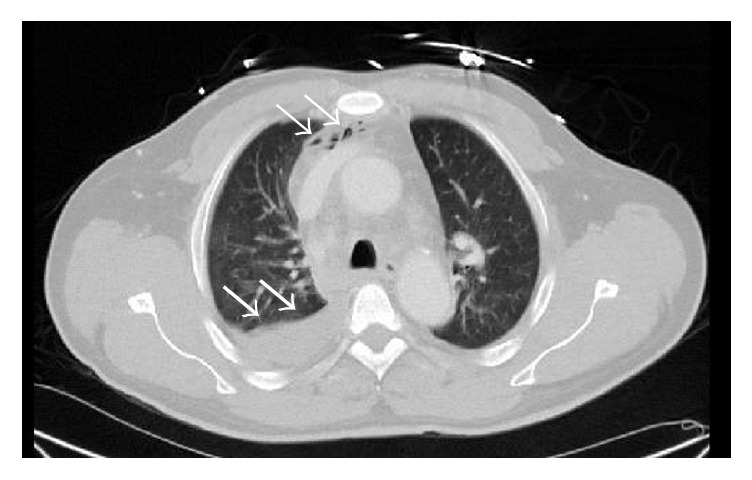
CT chest without contrast showed pneumomediastinum at the right superior mediastinum as shown by the white narrow arrow. The white thick arrow shows moderate right pleural effusion with 80 Hounsfield units suggestive of internal hemorrhage/proteinaceous content.
